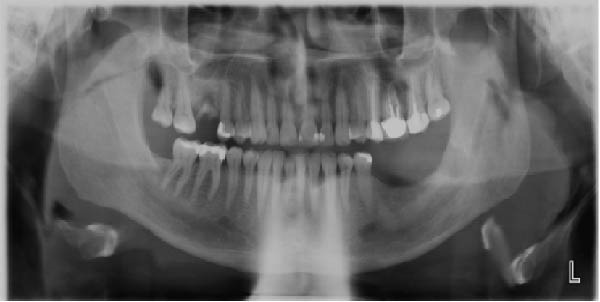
This is the postextraction orthopantomogram (OPG). A patch of radiolucency inferior to LL7/8 was seen.
